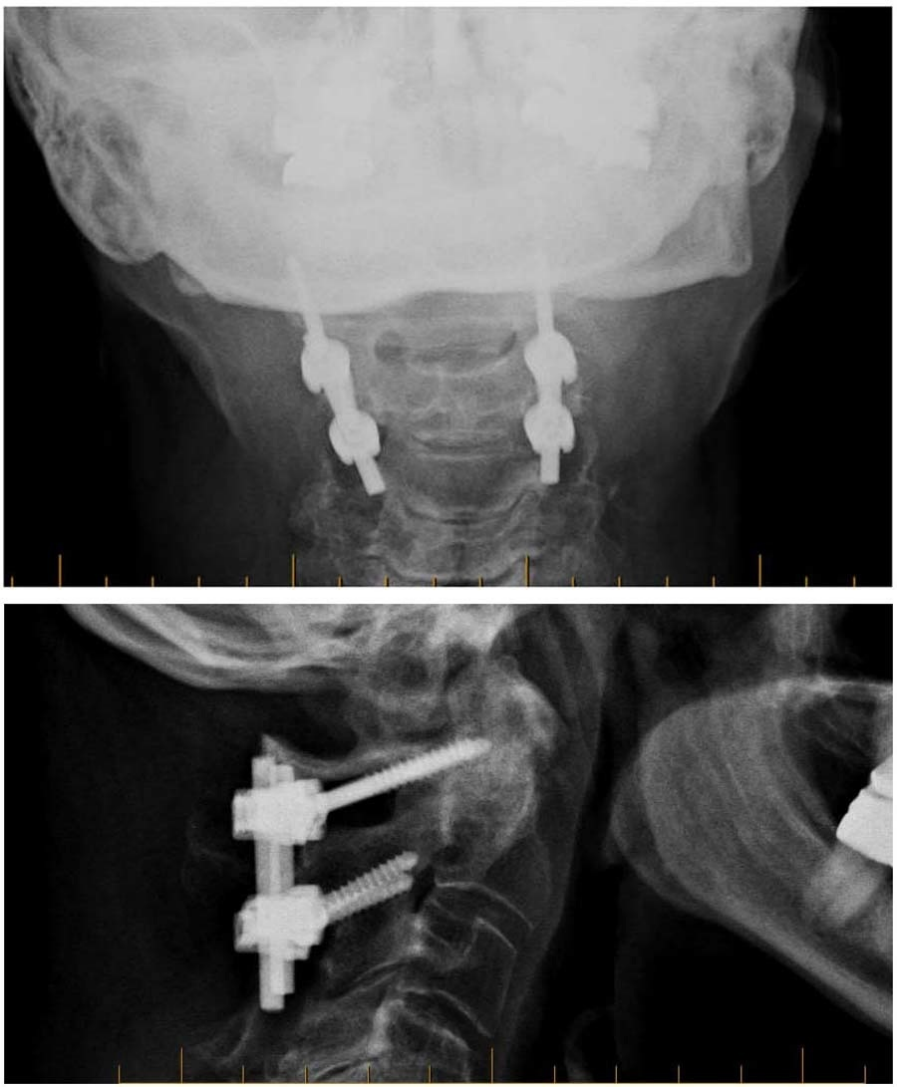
Postoperative images (X-rays)
